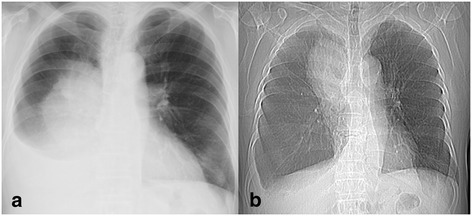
a Chest radiograph showing a large tumor shadow in the middle lung field and pleural effusion in the right cavity. b Scout image showing a large tumor shadow in the upper lung field. Note the change in position of the tumor
